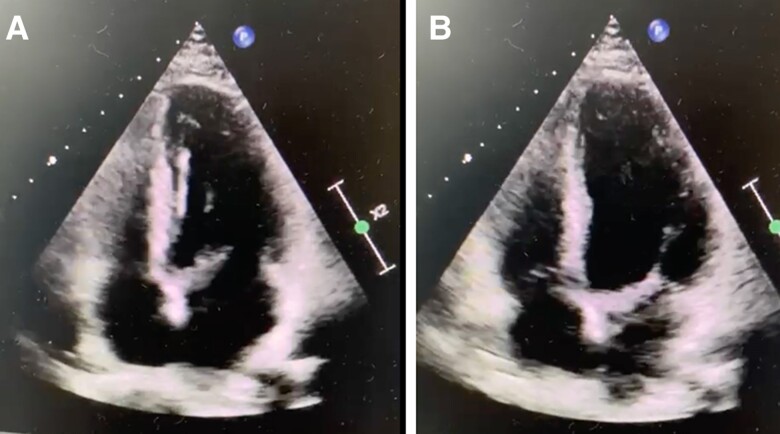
Echocardiographic images in a diastolic frame showing a thickened anterior mitral leaflet in diastole (*A*) and on the right side a systolic frame (*B*).
